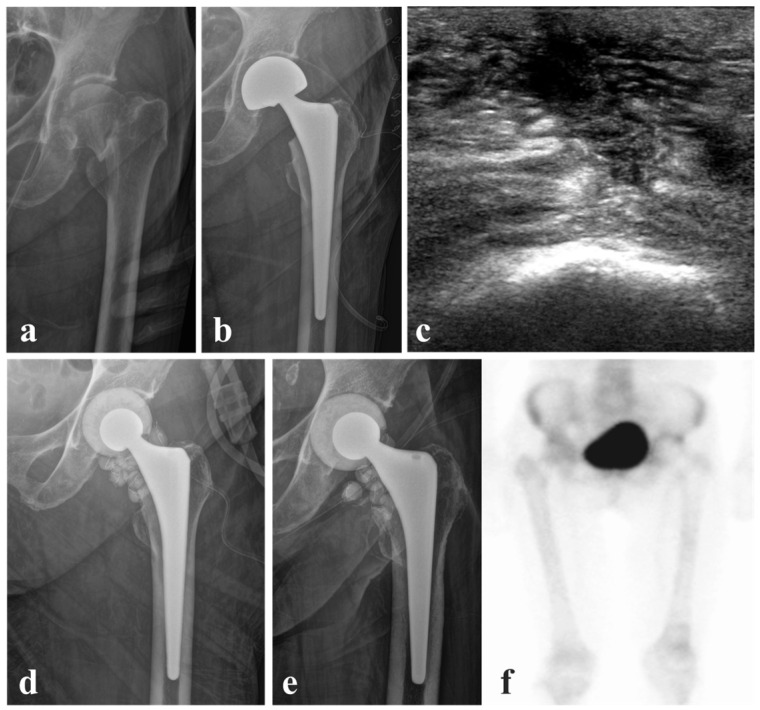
A case of a 78-year-old patient treated with partial two-stage revision that was discontinued after the first revision. (a) Preoperative anteroposterior hip radiograph showing a femoral neck fracture after a fall. (b) Postoperative radiograph after bipolar hemiarthroplasty. (c) Sonographic image obtained before aspiration, suggestive of PJI. (d,e) Immediate postoperative and 2-year follow-up radiographs after the first-stage revision with femoral stem retention. (f) Bone scan image obtained 2 years after surgery, showing negative uptake around the left hip joint.
